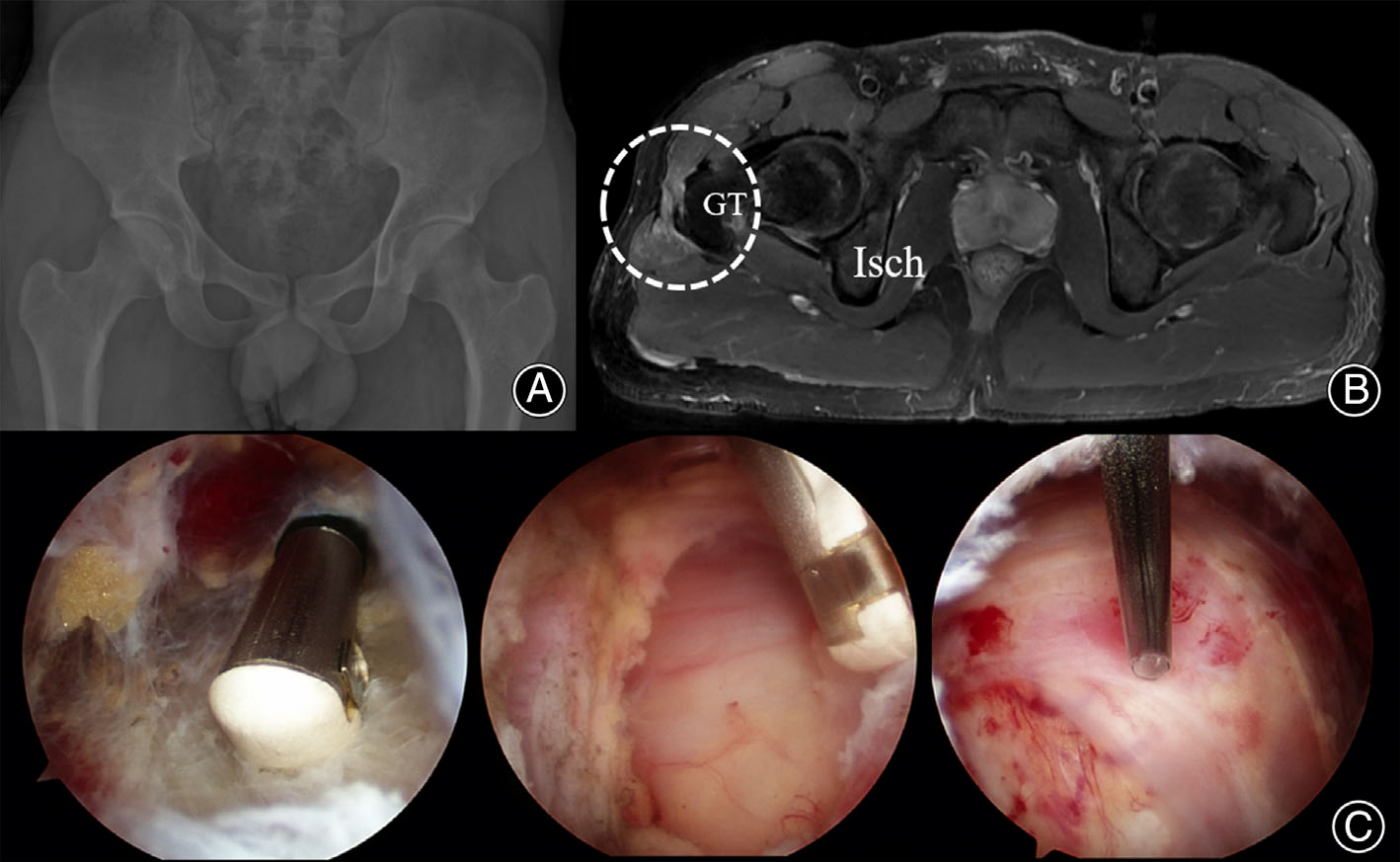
The pre‐/post‐operative images and arthroscopic finding of 20‐year‐old male patient who underwent arthroscopic iliotibial band release. The simple hip X‐ray covers the symptomatic region (A), the T_2_‐weighted magnetic resonance image show the signal change between iliotibial band and GT on right hip (B, circle), and arthroscopic images show maximal arthroscopic resection with GT bursectomy (C), but the snapping was not completely resolved. GT, greater trochanter.
